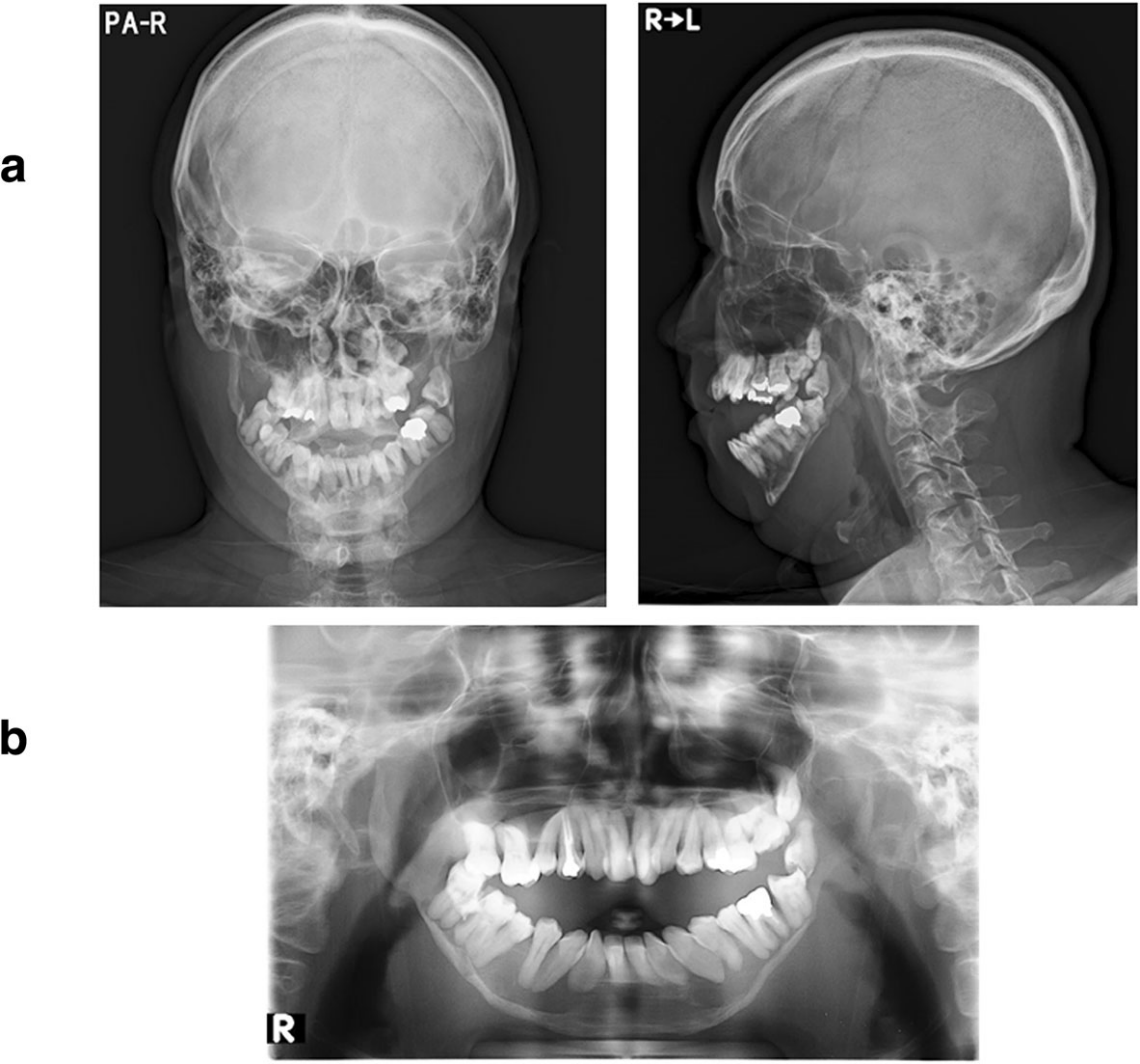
X-ray images. a Posteroanterior and lateral skull views do not reveal morphological abnormalities of the head or face height and width. b A panoramic radiographic image shows axial and coronal thinning of the alveolar bone in the maxilla and the entire mandibular (condyle, angle, body, and alveolar) bone
